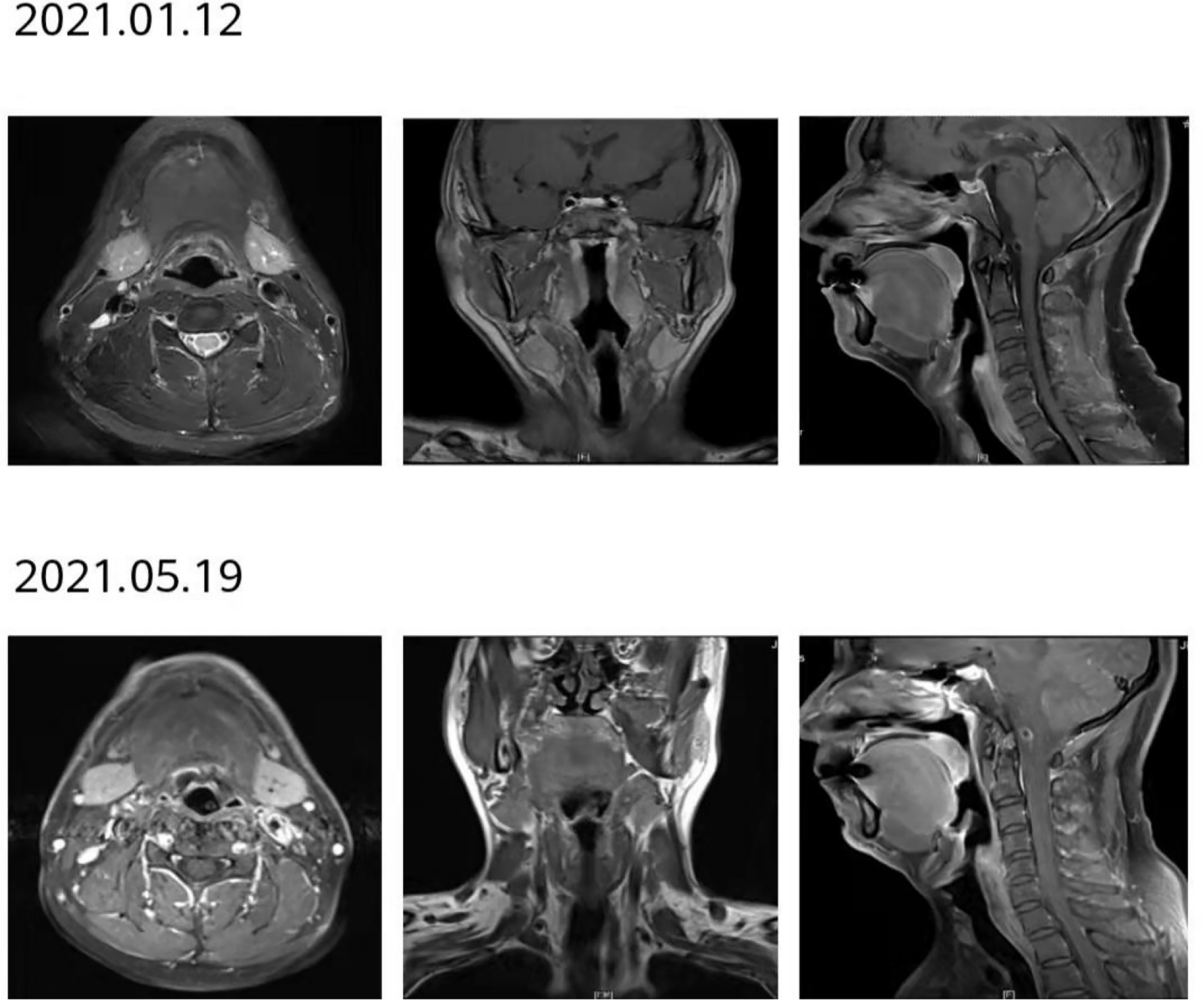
After 12 courses of treatment, no lesions were found in the reexamination of throat MRI. Compared with that after 8 courses of treatment, the curative effect was evaluated as maintaining CR.
